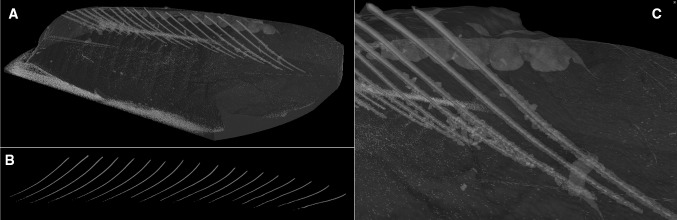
A typical sample captured by a micro X-ray CT scanner (trout 4–5 k) for volume analysis. a A section of a trout fillet with bone highlighted, b bones in situ with flesh removed, c closer examination of the bones within the flesh of the fish
